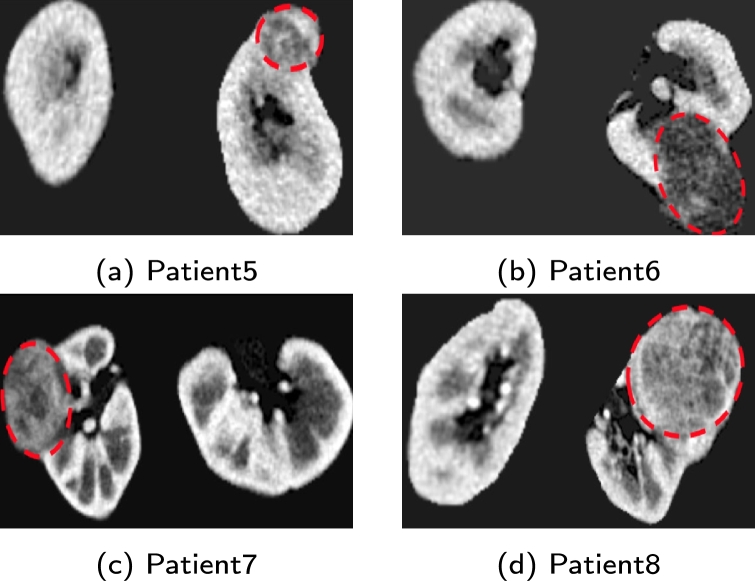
CT images with tumors indicated of patients with event 0.
